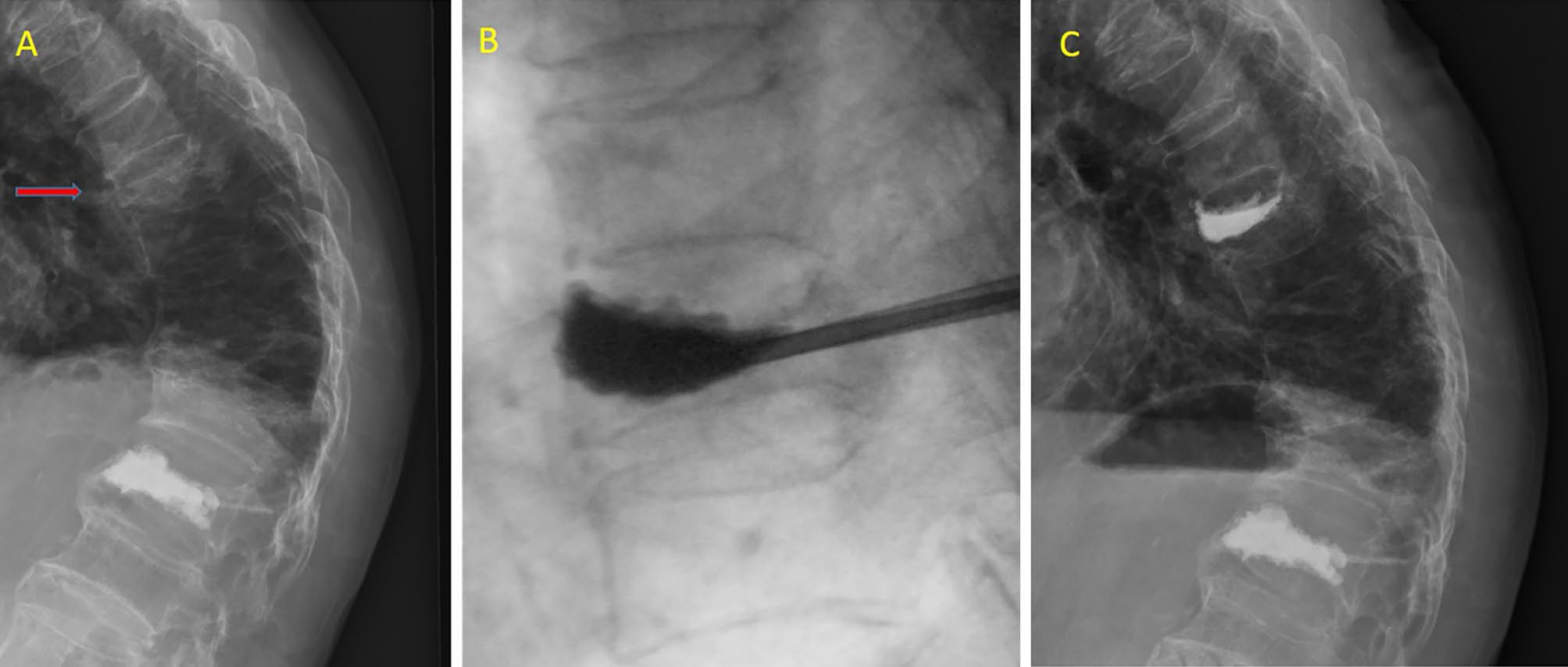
A case of T8 vertebral compression fracture treated with high-viscosity cement vertebroplasty. (A) The arrow in the preoperative lateral radiograph points to the fracture level. (B) Intraoperative radiograph. (C) Postoperative lateral radiograph shows fractured vertebrae filled with high-viscosity cement.
